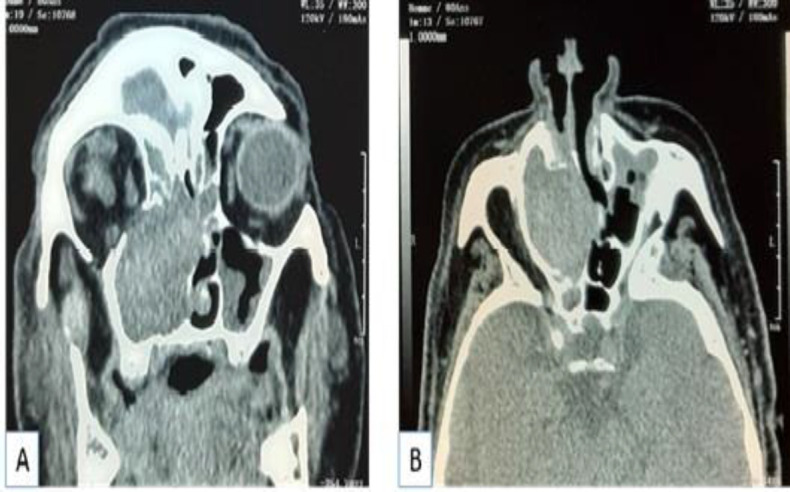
Nonenhanced CT of the paranasal sinuses: coronal section (A) and axial section (B) show a well-defined expansible mass of the right maxillary sinus, the frontal sinus, the ethmoid and the nasal cavity with destruction of the medial wall of the sinus, the nasal septum and the orbital floor.
